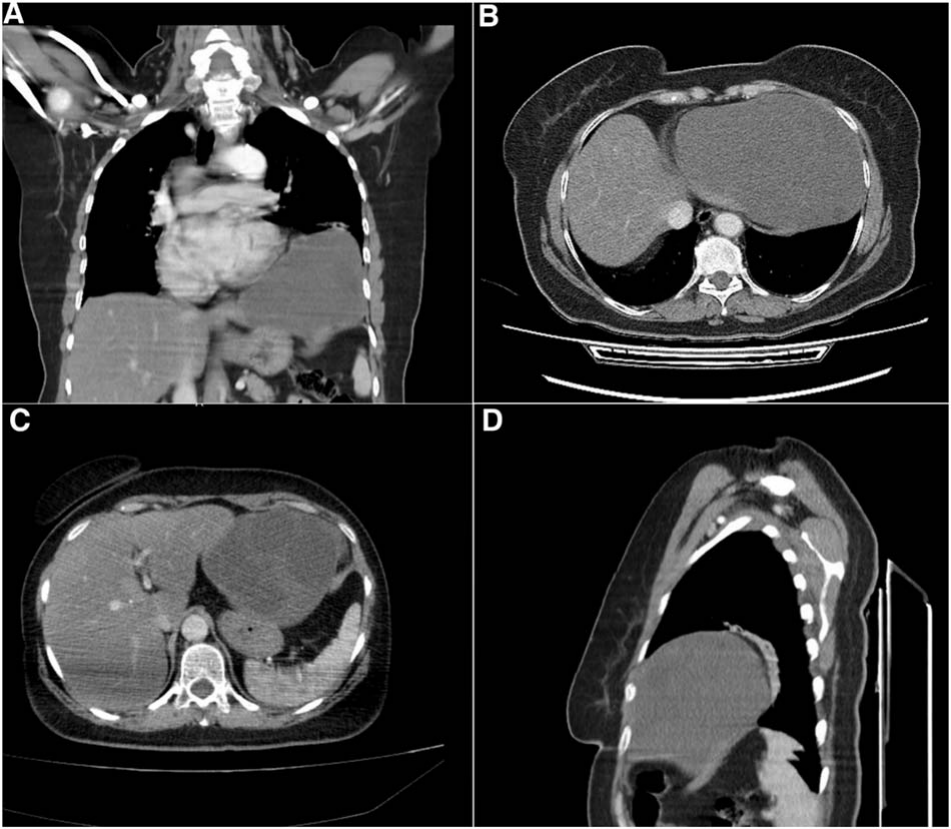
Computed tomography (CT) scan. (A) Coronal section, shows the cystic formation in the left hemi-thorax. (B) and (C) Axial section, shows the borders with the pericardium and the left hepatic lobe. (D) Sagittal section, shows the limited posterior extension of the cyst.
